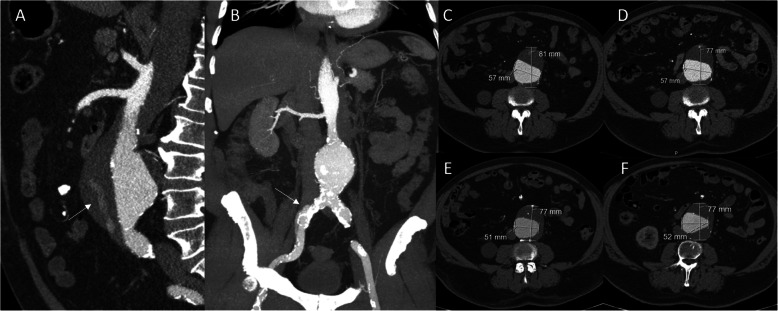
Preoperative CT angiography of the post-dissection infrarenal aneurysm in the sagittal plane showing the filling of the false lumen (arrow) (a), coronal maximum intension projection (MIP) reconstruction (b) and transversal slides of a CT angiography of the post-dissection infrarenal aneurysm before treatment (c), 1 month postoperatively (d), 8 months (e) and 15 months (f) postoperatively
